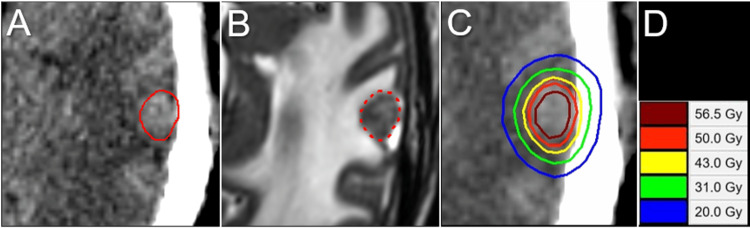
Case 2: Target definition and dose distribution for five-fraction stereotactic radiosurgery The images show planning axial CT (A, C); axial T2-WI (B); the GTV contour superimposed on the images (A, B); dose distribution (C); and representative isodoses (D). (B) A solid mass lesion mainly involved with the cortex in the left temporal lobe. CT: computed tomography; WI: weighted image; GTV: gross tumor volume
